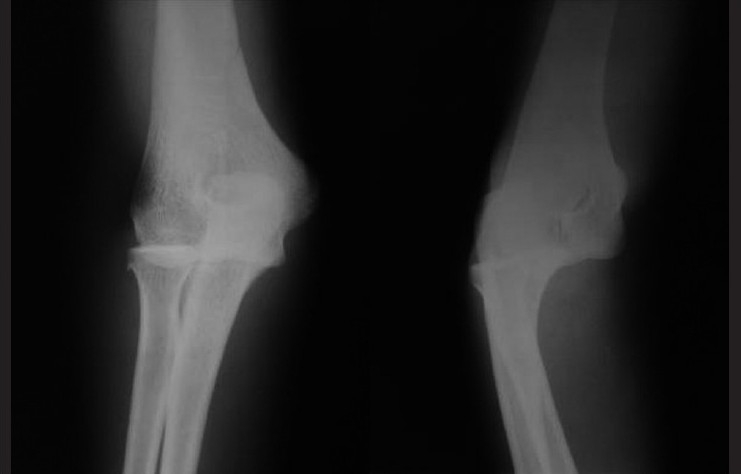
Radiographs of the elbow showing a posterior dislocated radial head
